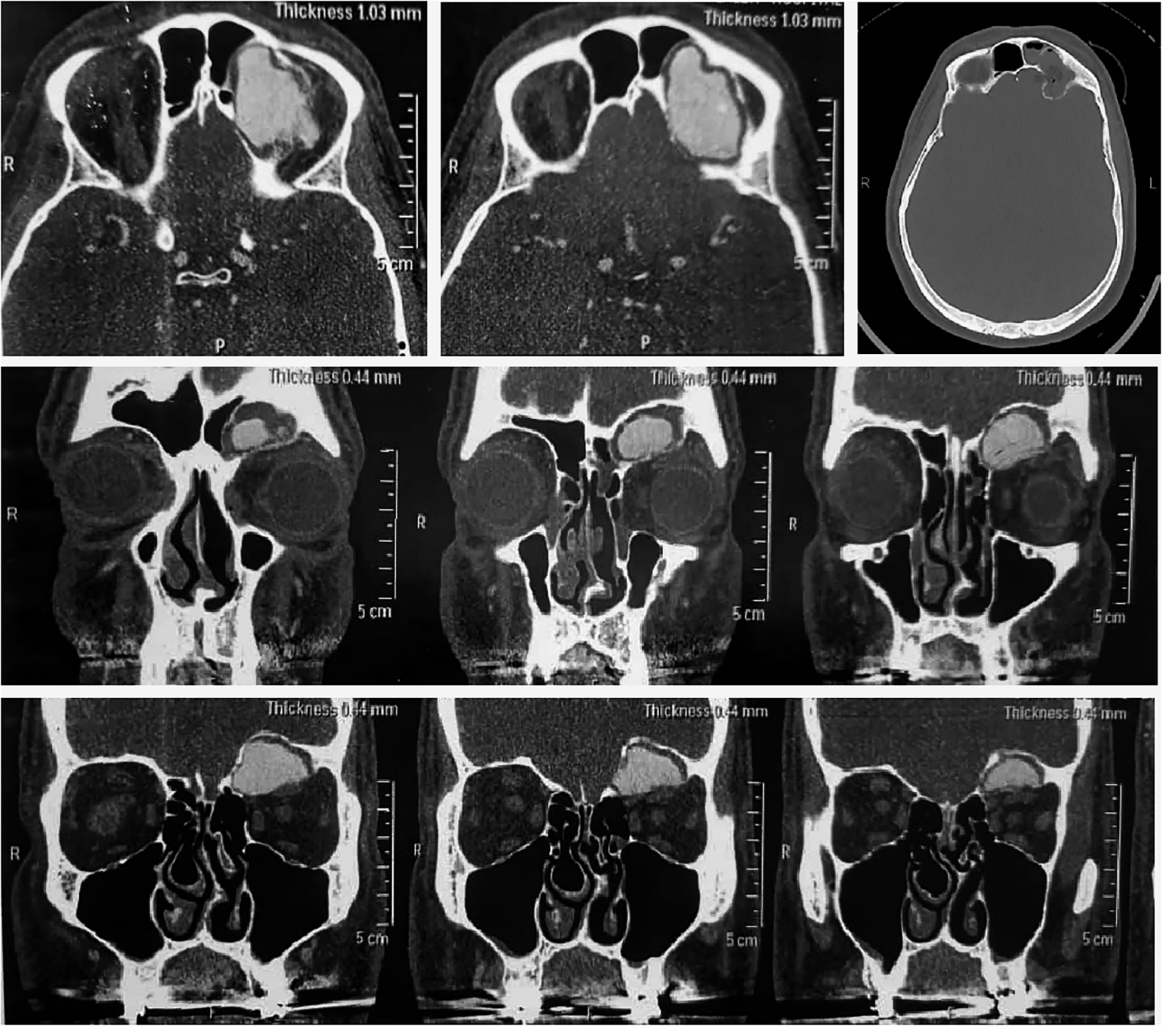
On the axial and coronal sequences of the CT scan with contrast, there is a well‐defined lytic expansile growth pattern with psammomatoid calcifications as small, round calcification in peripheral region of tumor in the left frontal sinus. There is no evidence of sclerotic margins or invasive features, cortical breakthrough. The images show significant contrast enhancement that is proposed the vascularity nature of the tumor.
